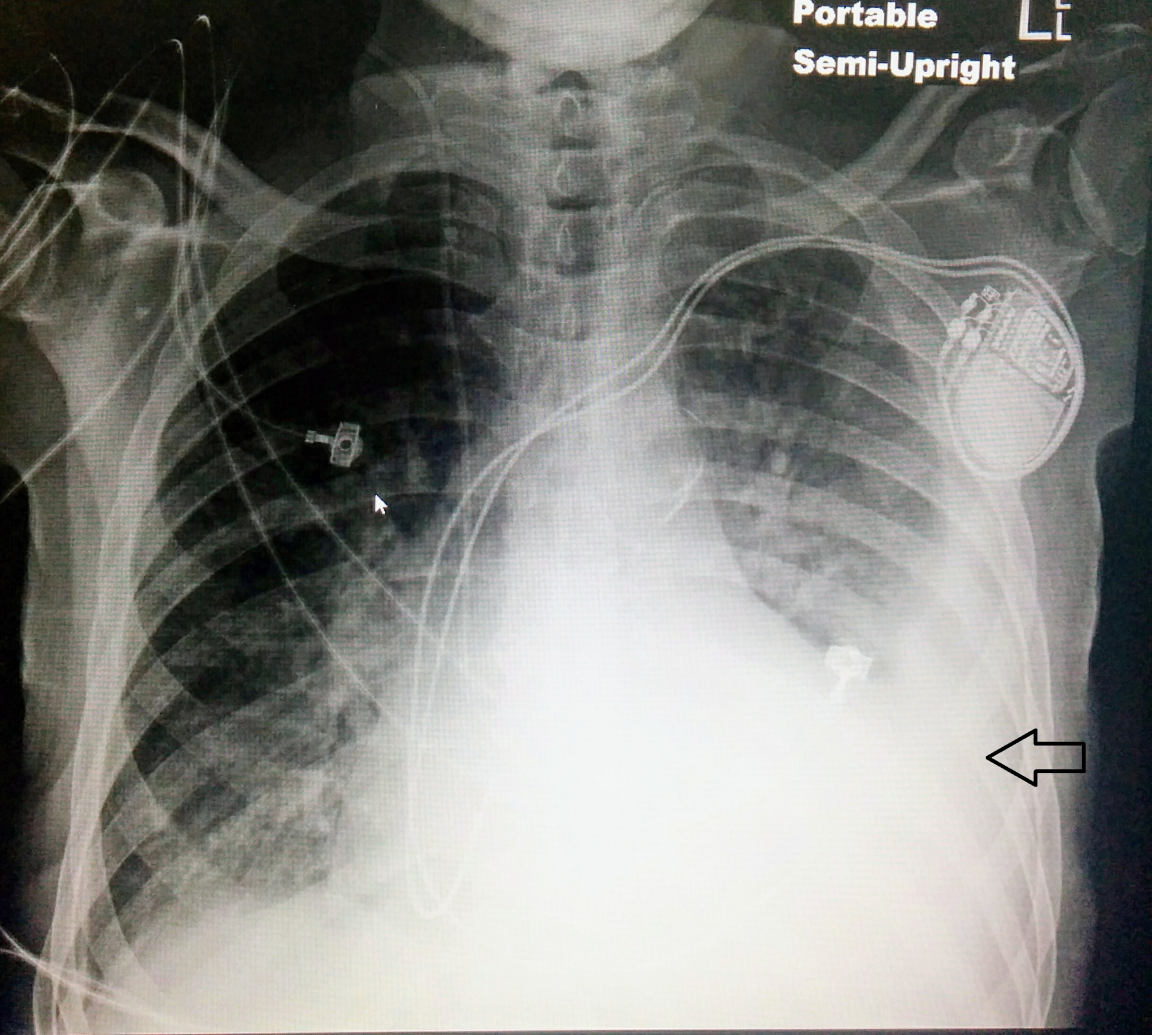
Portable chest X-ray (AP view) showing left sided pleural effusion (arrow) and vascular congestion AP - anteroposterior.
